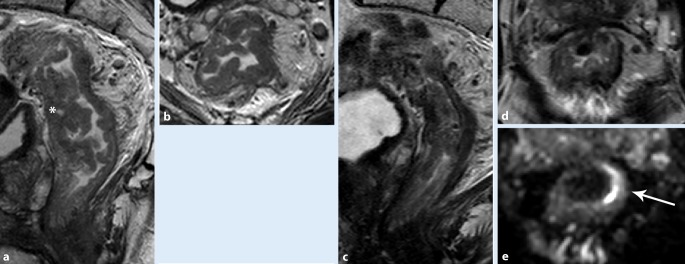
Magnetic resonance imaging (MRI) of a male patient before (a, b) and after chemoradiotherapy  (c, d) shows locally advanced rectal cancer (*white asterisk*) that responded poorly to the neoadjuvant chemoradiotherapy. Restaging MRI (c, d) shows a residual (isointense) mass (e; *white arrow*), and on diffusion-weighted imaging a restricted diffusion in the local tumor bed (e)
